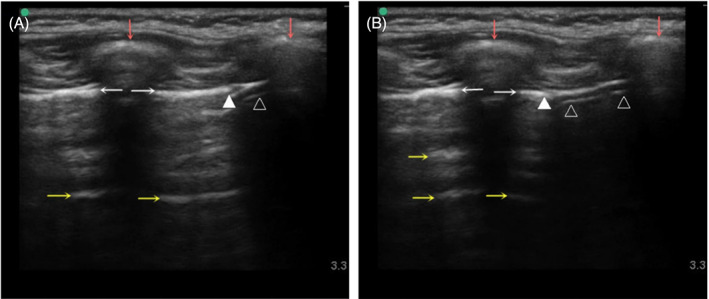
Curtain sign. (A) Ultrasound image at the end of inhalation. (B) Ultrasound image at the end of exhalation. Red arrow: rib; white arrow: pleura; yellow arrow: lung air artifact. Solid arrow: lung base; Open arrow: diaphragm. The overlap of the costophrenic recess onto the abdomen creates a demarcated leading boundary of the lung air artifact. With the breathing movement, the lower boundary of the lung moves back and forth in the cephalo‐sacral direction at the costophrenic recess, giving the impression of a lung curtain
